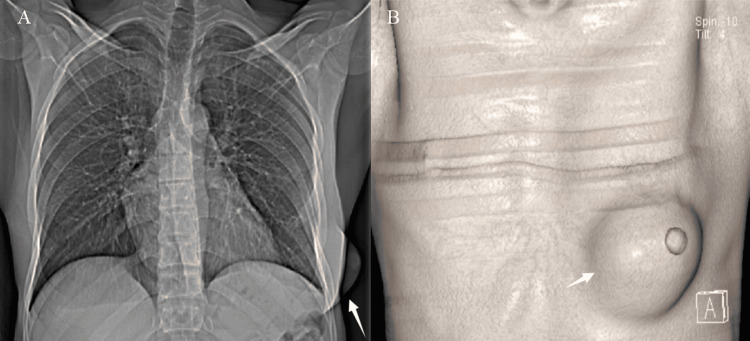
Chest X-ray and CT scan reconstruction demonstrating left breast soft tissue expansion. Image (A) is a posteroanterior (PA) chest X-ray revealing thickening and swelling of the soft tissues of the left thoracic wall with breast asymmetry (white arrow) and a slight diffuse increase in peribronchovascular markings. Image (B) is a chest CT with a 3D reformat demonstrating the significant expansion of the left breast, lifting the nipple (white arrow).
